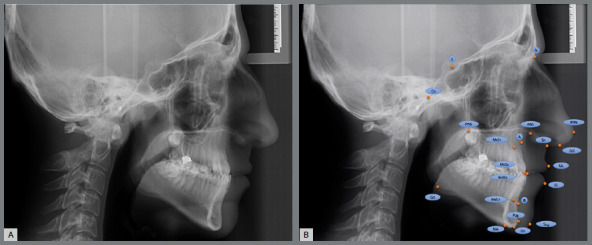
A) Lateral cephalometric radiograph. B) Cephalometric landmarks. S= Sella, N= Nasion, Co= Condylion, ANS= Anterior Nasal Spine, PNS= Posterior Nasal Spine, PRN= Pronasale, A= A point, B= B point, Sn= Subnasale, Col= Columella, Mx1r= Maxillary first incisor root, Mx1c= Maxillary first incisor crown, UL= Upper Lip, LL= Lower Lip, Md1c= Mandibular first incisor crown, Md1r= Mandibular first incisor root, Go= Gonion, Pog= Pogonion, Me= Menton, Gn= Gnathion, SPog= Soft tissue pogonion.
